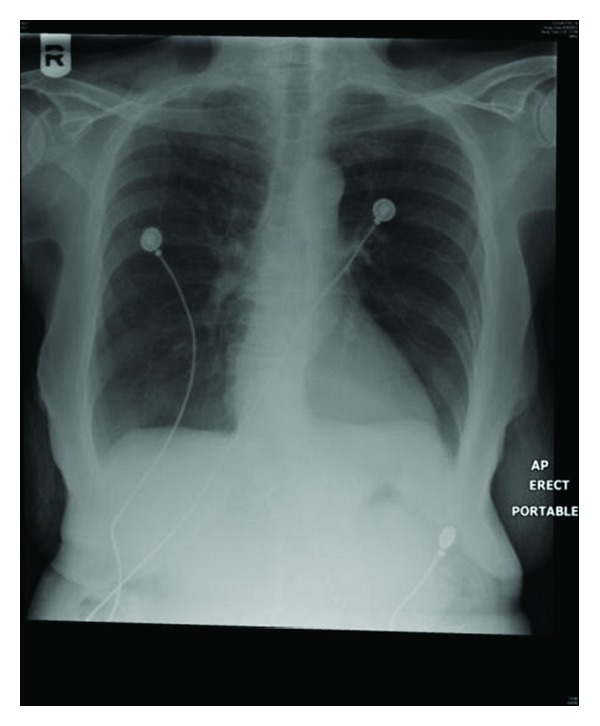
Preoperative chest X-ray: essentially normal.
